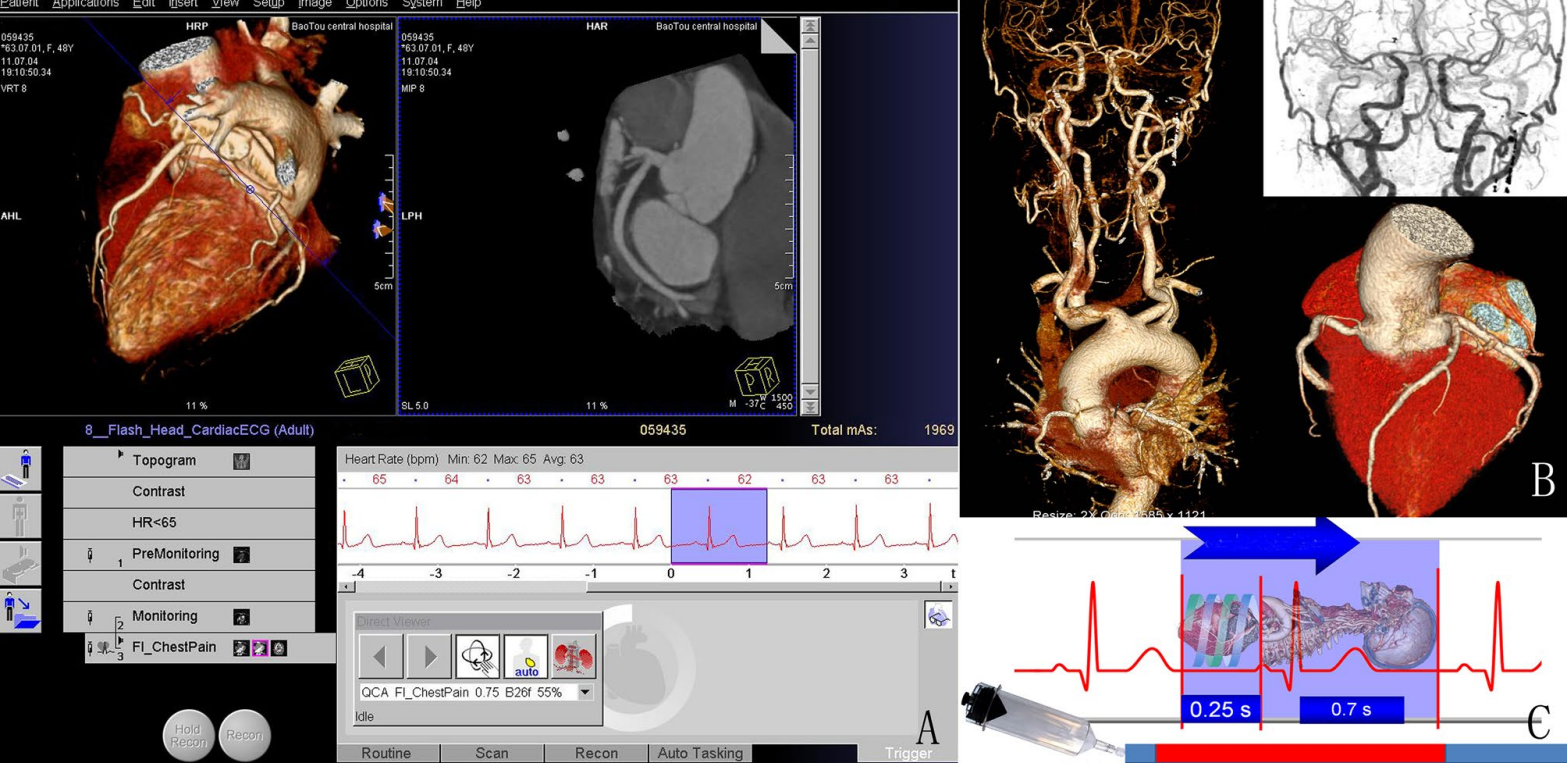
Operator interface (A) and typical image (B) of the high-pitch protocol for one-step ICCC-CTA. Scan direction and the relationship between contrast agent injection time and scan time with the ICCC-CTA protocol (C). ICCC-CTA, Integrated coronary–carotid–cerebral computed tomography angiography
